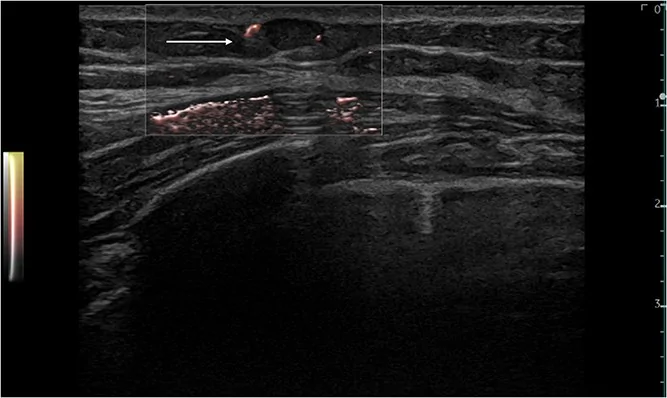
The color-Doppler ultrasonography of the breast revealed a subcutaneous, fairly well-defined, hypoechoic lobular lesion, with posterior acoustic enhancement, measuring 7.5 x 6.5 x 4.5 mm with low internal blood flow (arrow).
